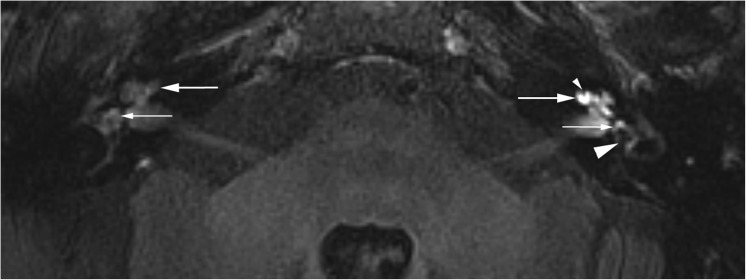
Axial delayed gadolinium-enhanced 3D FLAIR images at the level of the inner ear in a 77-year-old woman with unilateral left-sided definite MD and cochlear hydrops grade I (small arrowhead) and vestibular hydrops grade II according to the four-stage grading system (large arrowhead). Note increased vestibular (small arrow) and cochlear (large arrow) perilymphatic enhancement (PE) on the symptomatic side compared with the normal right labyrinth. This is the signature of BPB-impairment
